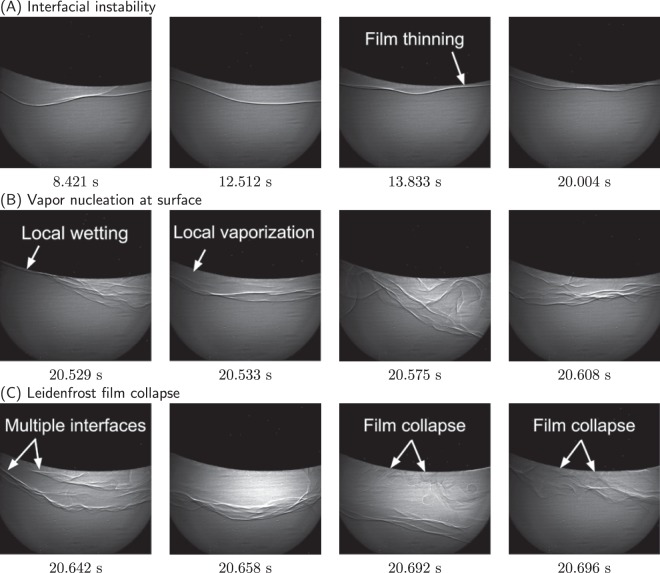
X-ray images of the Leidenfrost film collapse process for trial RO3 of the rough sphere. The brightness and contrast of each image has been enhanced for clarity. Image dimensions correspond to ~1914 *μm* × 1914 *μm*. (A) Interfacial instability. (B) Local wetting of the surface, followed by vapor nucleation and recovery of the liquid-vapor interface. (C) Leidenfrost film collapse.
